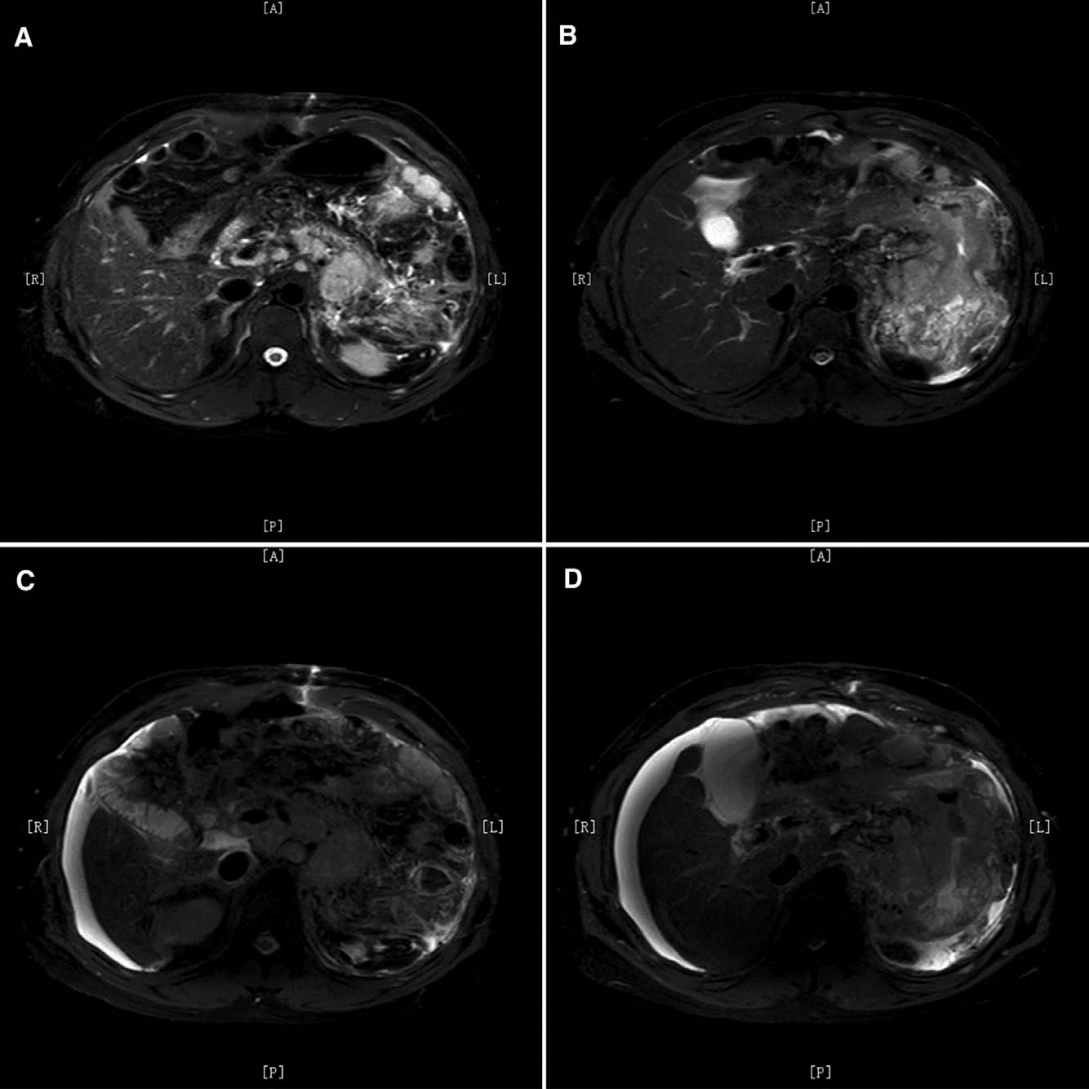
MRI before (A, B) and after (C, D) third line treatment with FLOT chemotherapy showed no change in the stomach wall lesions, significant advancement of multiple lymph node metastases, and unclear boundaries between enlarged lymph nodes and local structures.
